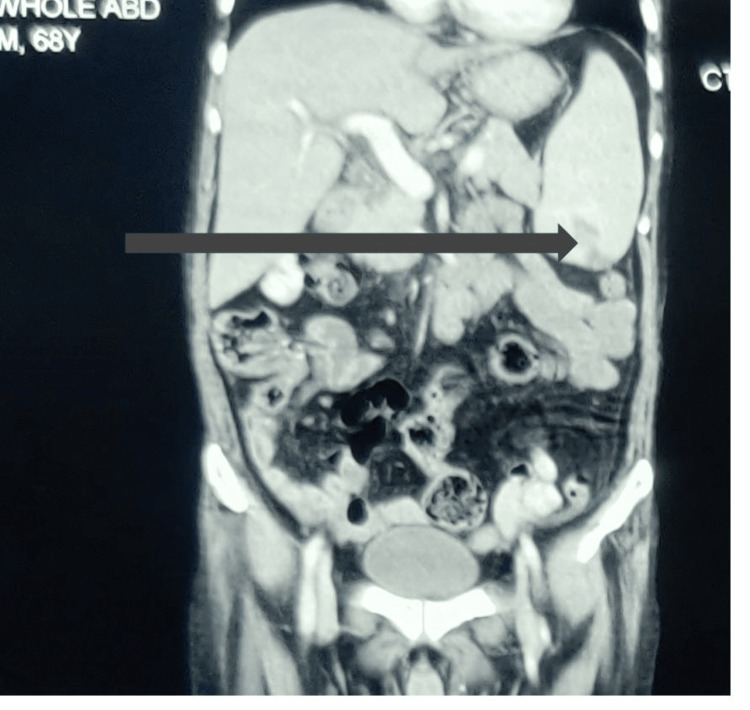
Contrast-enhanced computed tomography shows the presence of a hypodense wedge-shaped area without any contrast enhancement at the inferolateral pole of the spleen.
